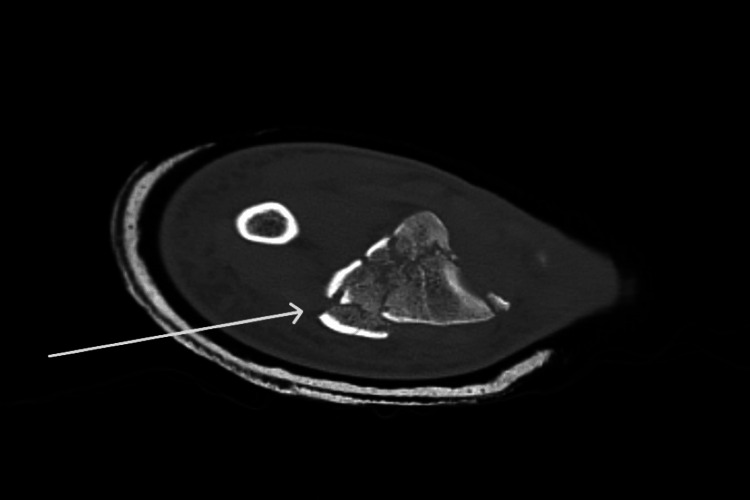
Admission CT X-rays of the right distal radius showing a comminuted intra-articular fracture with volar displacement. Arrows indicate fracture fragments.
